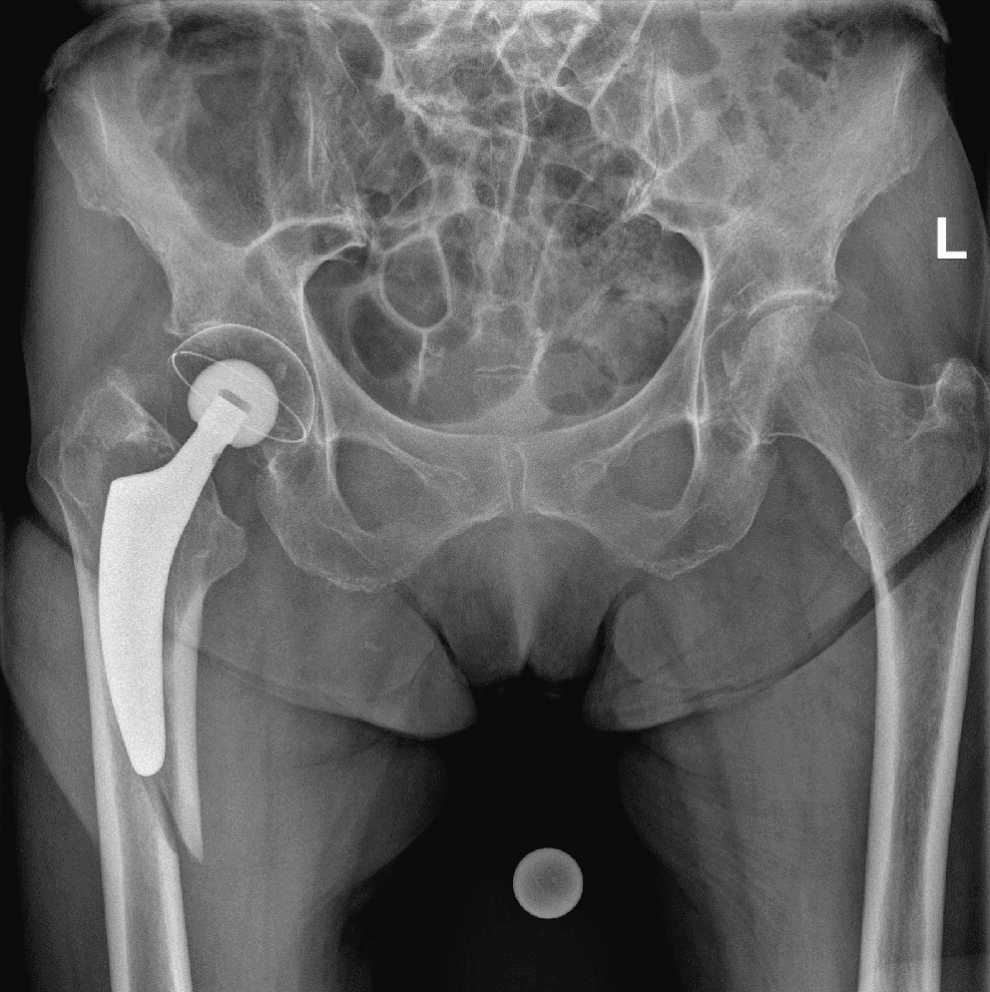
Radiograph of a right-sided Vancouver B2 fracture around a non-cemented hip arthroplasty with a short stem
